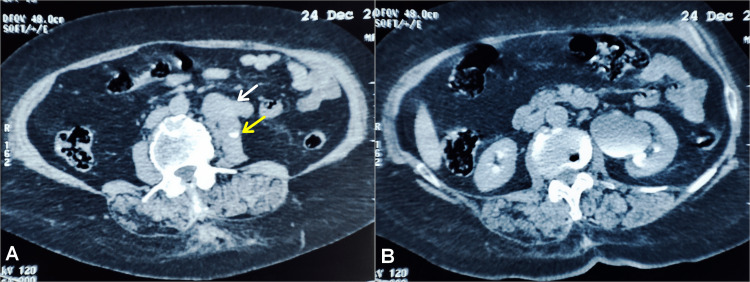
Axial contrast-enhanced CT of the abdomen showing a circumscribed, lobulated, and heterogeneously enhanced left retroperitoneal mass No evidence of necrosis or hemorrhage was observed in the tumor. There were neither calcified areas nor cystic components inside, and no signs of metastatic disease were noticed elsewhere. (A) Axial CT image showing the left ureter (yellow arrow) passing through the dorsal side of the mass (white arrow). (B) Axial CT image showing left hydronephrosis.
